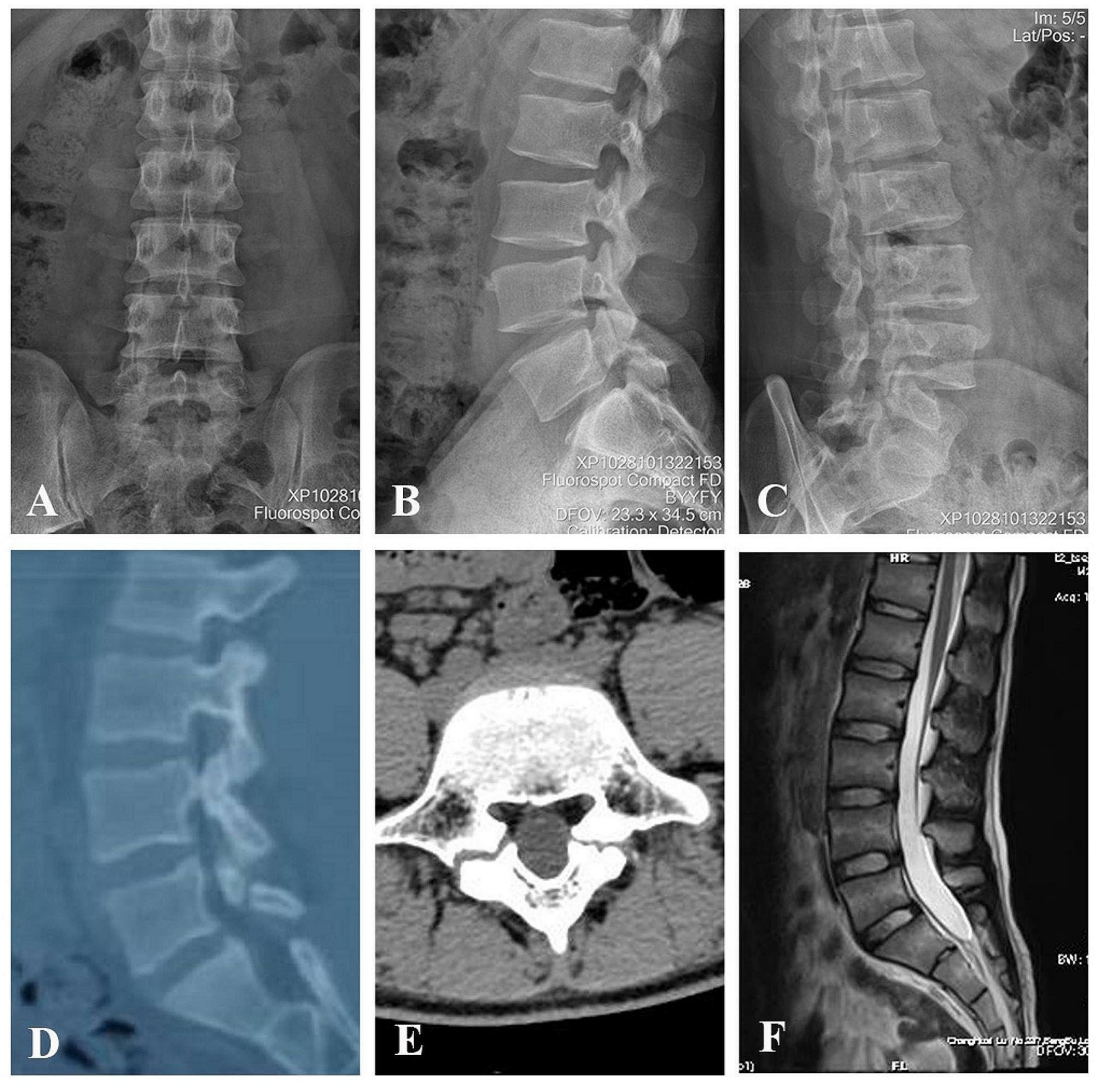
Case data Presentation. (A, B, C) X-ray images. (D, E) Computed tomography (CT) images. (F) Magnetic resonance imaging (MRI) pictures
